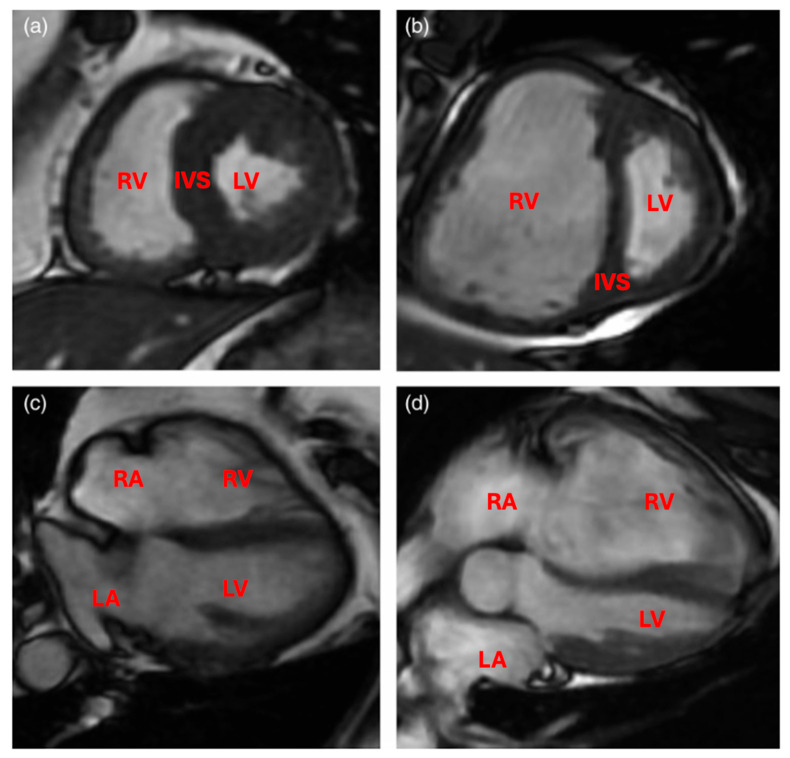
Cardiac MRI study of a patient with and a patient without pulmonary hypertension (PH). (a,c) Short-axis and 4-chamber view of a patient without PH; (b,d) Short-axis and 4-chamber view of a patient with PH. In patients with PH, elevated RV pressure causes RV hypertrophy and leads to increased septal angle deformity. RV = right ventricle; IVS = interventricular septum; LV = left ventricle; RA = right atrium; LA = left atrium; MRI = magnetic resonance imaging. Reprinted/adapted with permission from Ref. [86].
